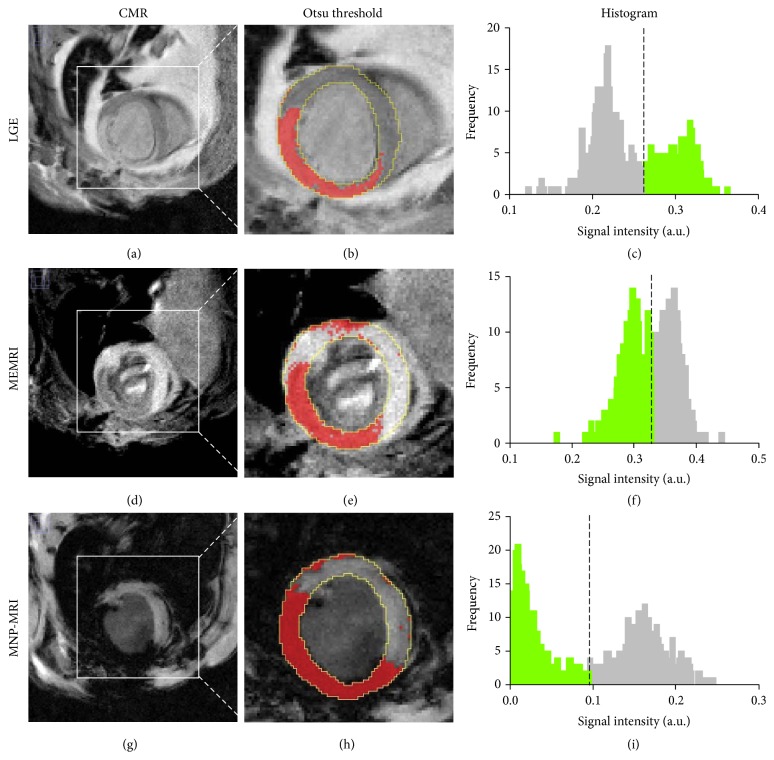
Area-based approach for contrast-enhanced size measurement. Enhanced area (red color) was demarcated using Otsu's threshold method (b, e, h). The epicardium and endocardium are traced in yellow. The dotted lines in the histogram correspond to the estimated threshold values and green bars represent enhanced pixels in MRI images (c, f, i). The sizes of enhanced area for LGE, MEMRI, and MNP-MRI was 38.7%, 46.3%, and 50.4%, respectively. The horizontal axes represent normalized signal intensity in the MR image.
